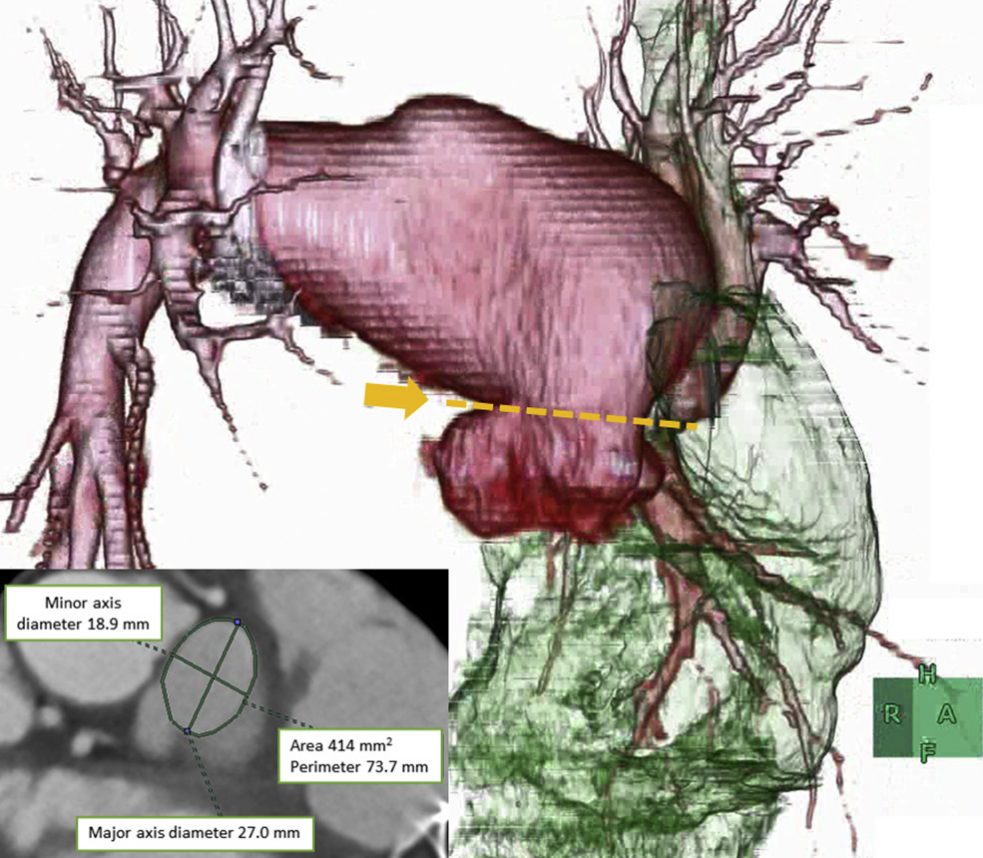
A 3-dimensional computed tomography image showing the pulmonary artery in a systolic phase from a right anterior oblique angle of 30 degrees, which revealed a significant constriction (yellow arrow) and subsequent dilation of the main pulmonary artery. The measured values of the constricted part were as follows: area, 414 mm^2^; perimeter, 73.7 mm; major axis diameter, 27.0 mm; and minor axis diameter, 18.9 mm.
